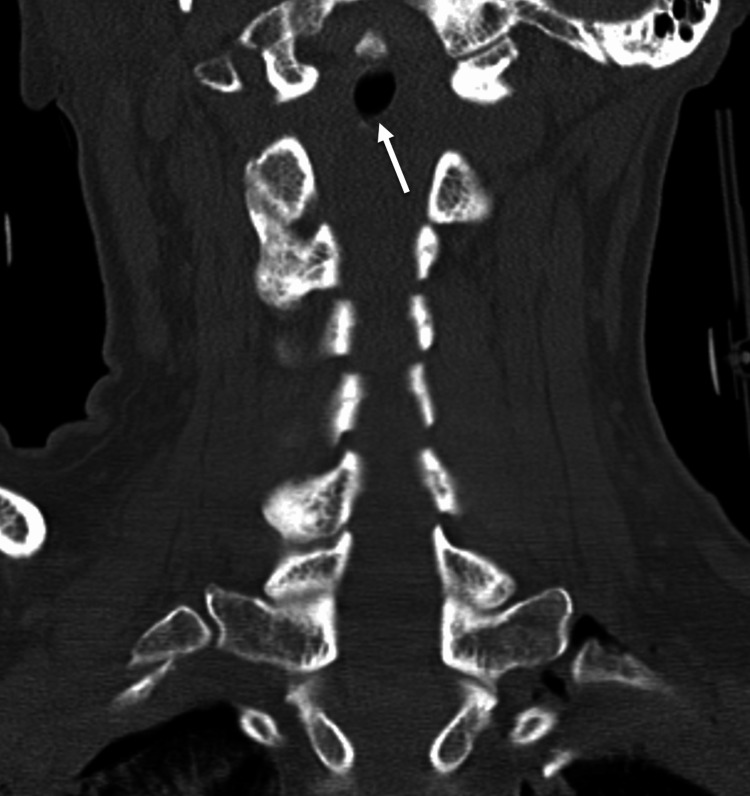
Coronal computed tomography of the cervical spine demonstrating intradural gas (arrow) and gas adjacent to neuroforamina.
